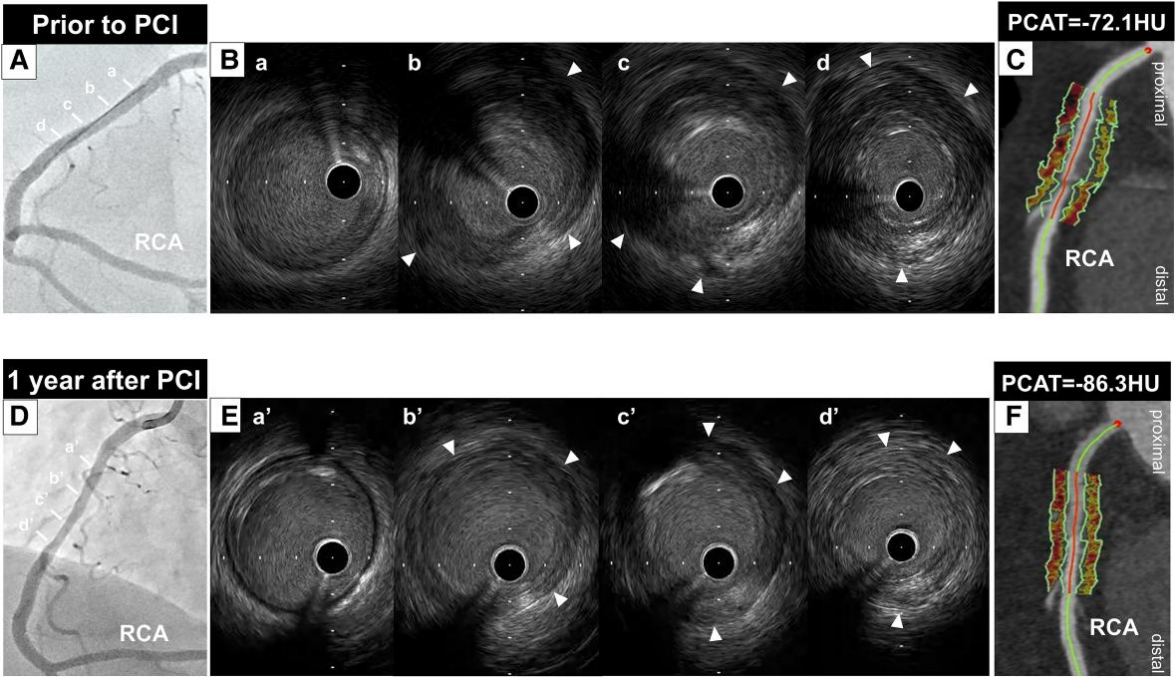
(*A*) Mild stenosis was identified in the proximal segment of the right coronary artery. (*B*) Intravascular ultrasound imaging revealed thickening of the adventitia (white arrowheads) at the proximal segment of the right coronary artery. (*C*) Coronary computed tomography angiography imaging 12 days after primary percutaneous coronary intervention demonstrated soft tissue proliferation surrounding the proximal segment of the right coronary artery. The average pericoronary adipose tissue attenuation at this segment was −87.1 HU. (*D*) Coronary angiography at 1 year after therapy did not show any progression of coronary artery stenosis. (*E*) A decrease in the thickness of the adventitia (white arrowheads) was observed on intravascular ultrasound imaging. (*F*) Coronary computed tomography angiography imaging demonstrated a reduction in soft tissue volume, and pericoronary adipose tissue attenuation decreased to −95.8 HU. HU, Hounsfield units; IVUS, intravascular ultrasound; PCAT, pericoronary adipose tissue attenuation; PCI, percutaneous coronary intervention; RCA, right coronary artery.
